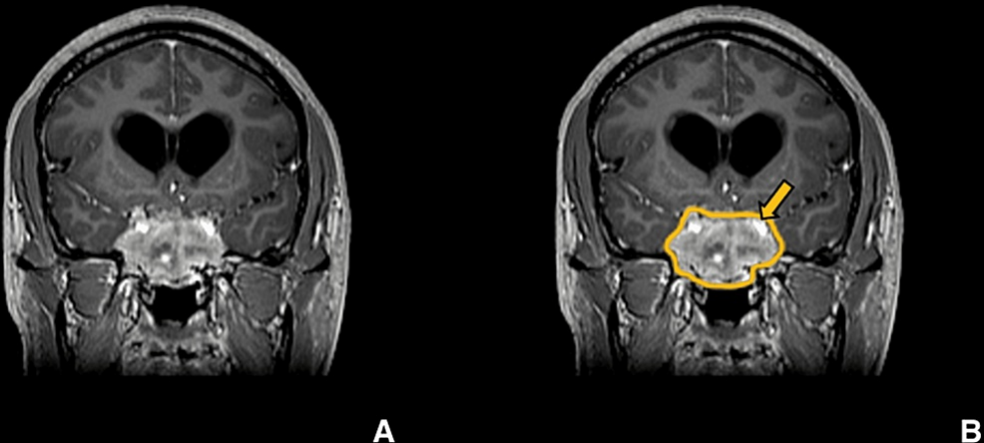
Preoperative imaging for patient 1. Contrast-enhanced T1-weighted coronal image showing sellar, suprasellar lesion with cavernous sinus invasion, which was more pronounced on the right side. (A) Without ROI, and (B) with ROI.
